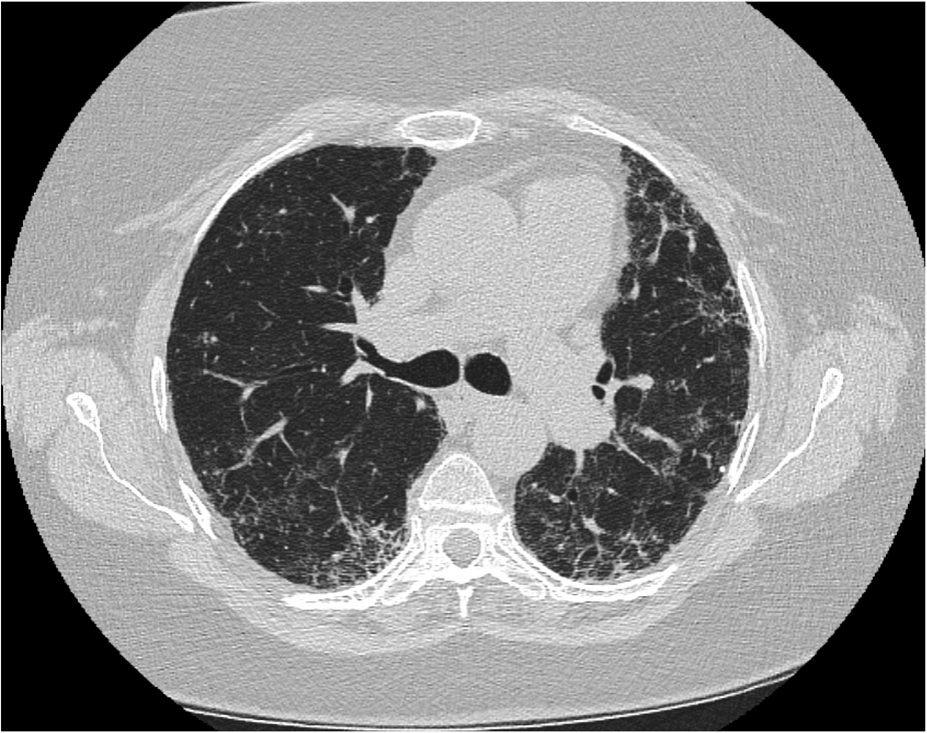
Computed tomography (CT) scan (axial section) showing fibrotic changes in the posterior and basilar part without any honeycombing.
